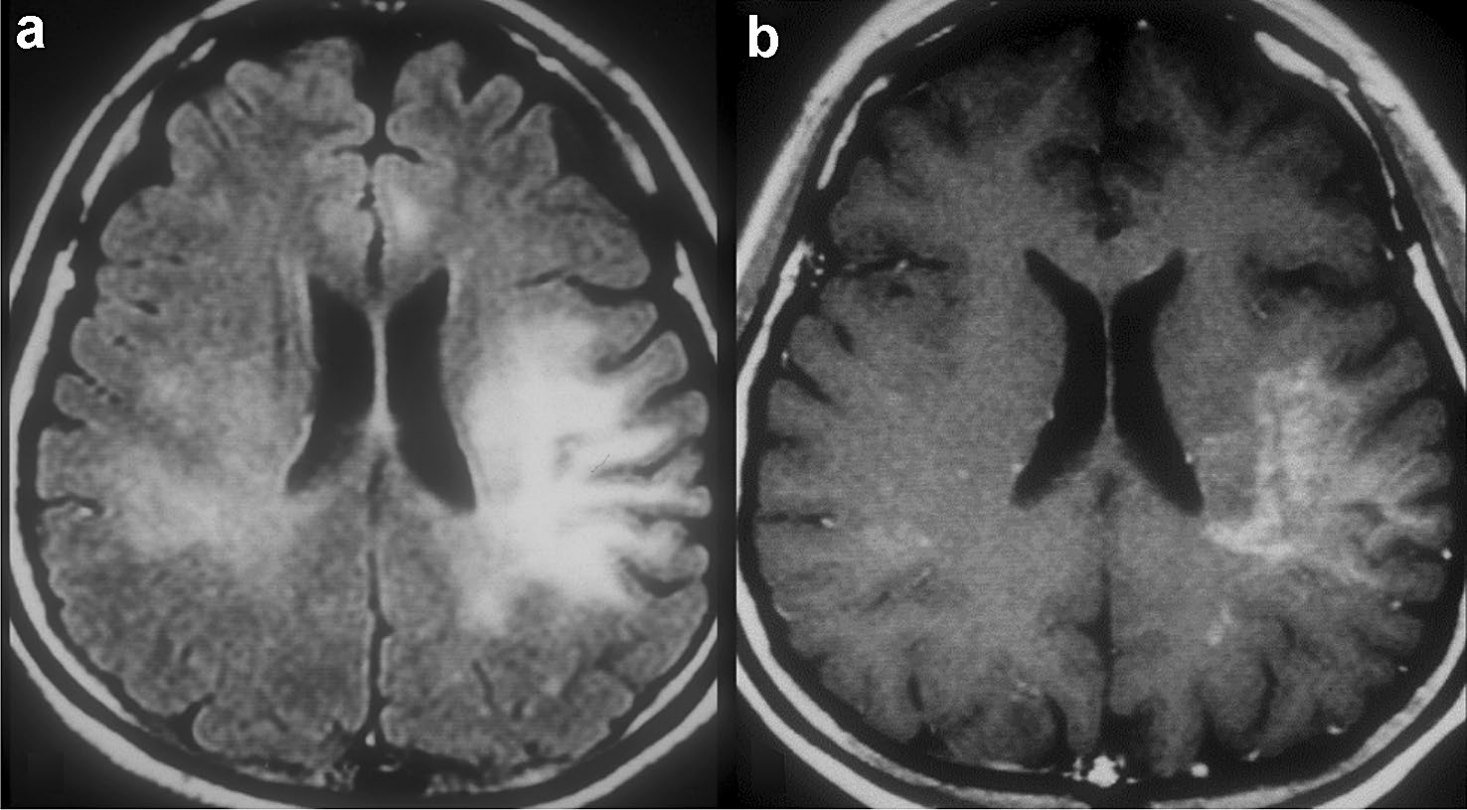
Immune reconstitution inflammatory syndrome (IRIS) developed in a patient with progressive multifocal leukoencephalopathy. FLAIR image (a) shows white matter lesions due to PML. Contrast-enhanced T1-weighted image (b) shows parenchymal and perivascular enhancement (b) compatible with IRIS
